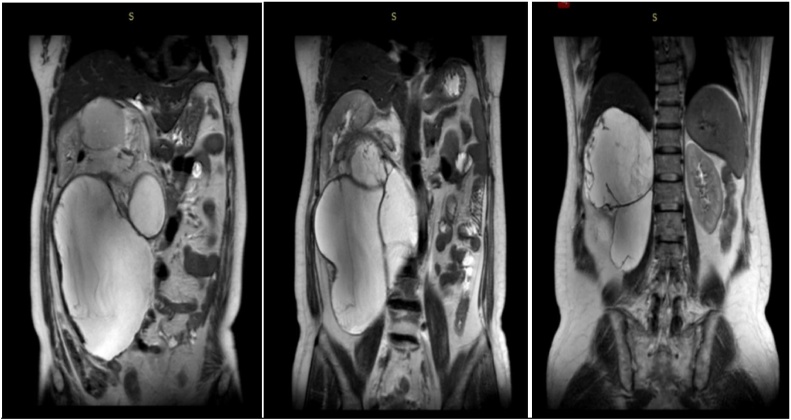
This coronal MRI of the abdomen shows a large retroperitoneal tumor. The organs have deviated from their normal position.
